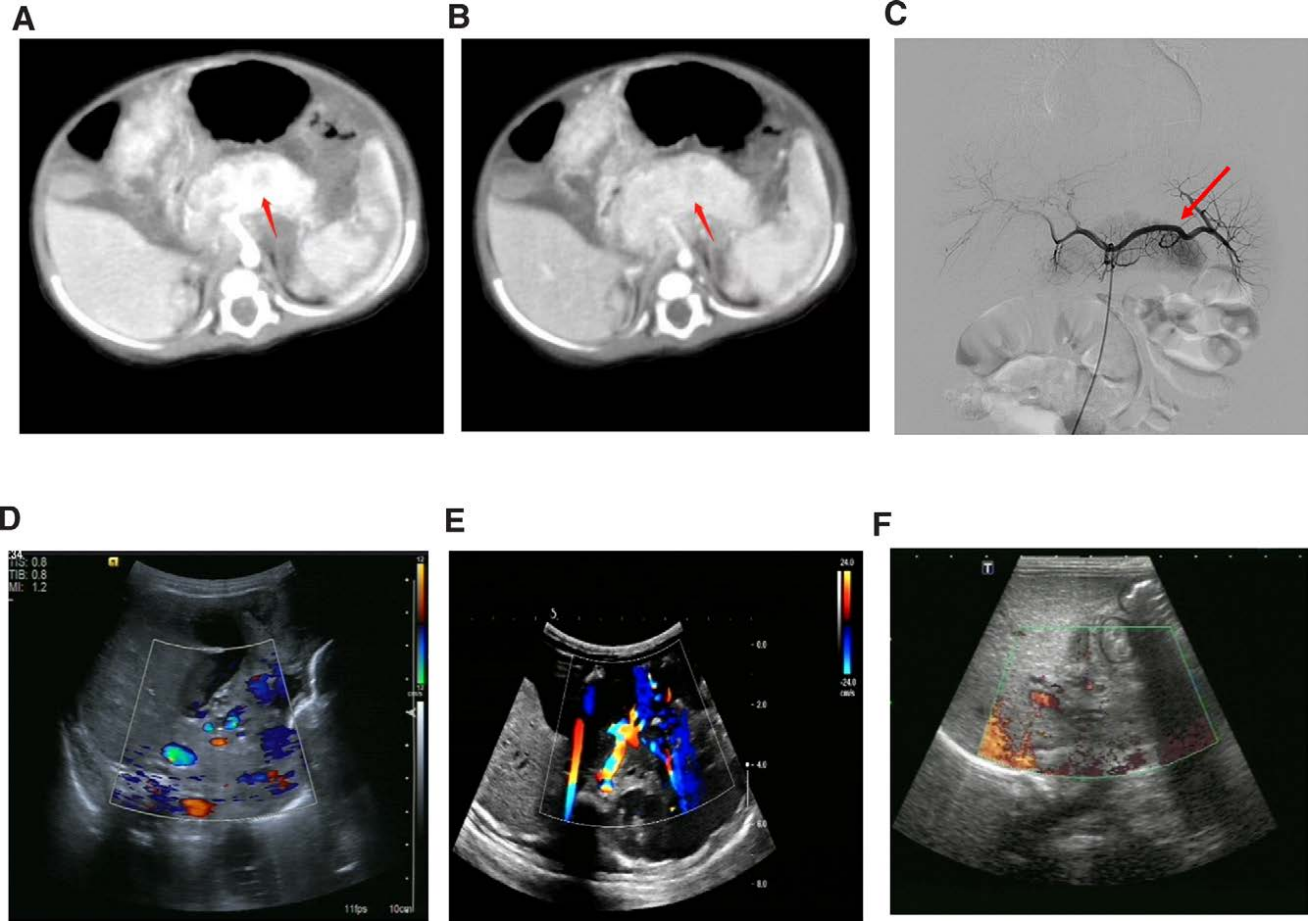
Representative clinical images of patient 7 before and after treatment. (A, B) Enhanced CT angiogram revealed a moderate enhancement in the retroperitoneum, demonstrated by the mass in the arterial phase and the venous phase at diagnosis. The arrow indicates the pancreas. (C) Based on the DSA result at diagnosis, there was no apparent tumor observed; however, there was a partial enhancement on the pancreatic tail. (D) Ultrasonographic findings of pancreatic hemangioma with increased peripheral echogenicity and hypoechoic central appearance. (E, F) No obvious arterial flow was detected after treatment in the pancreatic area. CT = computed tomography.
